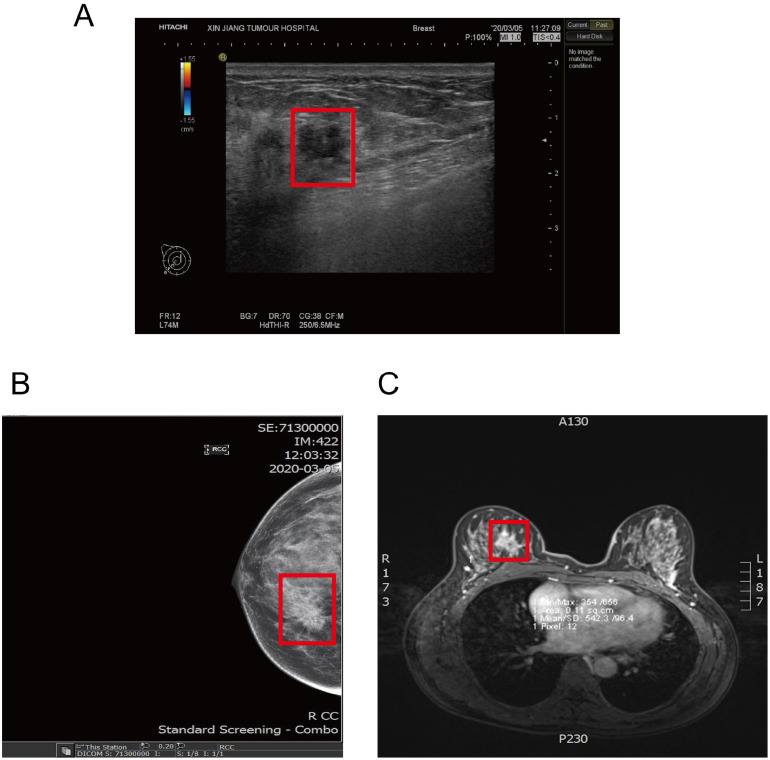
Initial diagnostic. (A) Color Doppler ultrasonography (March 5, 2020) showed an irregular solid hypoechoic mass in the upper inner quadrant of the right breast (3.3 × 2.4 × 2.3 cm, inside the red box), with indistinct margins and intralesional vascular signals. (B) Mammography (March 5, 2020) demonstrated an irregular high-density mass in the upper inner quadrant of the right breast (approximately 27 × 15 mm, inside the red box) with spiculated margins; nipple inversion was noted without obvious skin thickening. (C) Breast MRI (March 9, 2020)revealed an irregular spiculated mass in the upper inner quadrant (27 × 19 × 23.6 mm, inside the red box) with diffusion restriction and heterogeneous rim enhancement with central non-enhancing areas; the time–intensity curve showed an early enhancement–plateau (type II) pattern.
